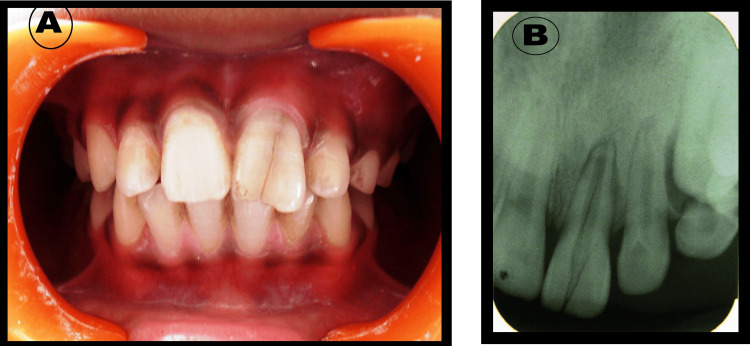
a) Maxillary left central incisor presented with vertical crown fracture. b) IOPA showing two longitudinal vertical fracture lines extending from the crown to the root portion of 21 IOPA: intraoral periapical
